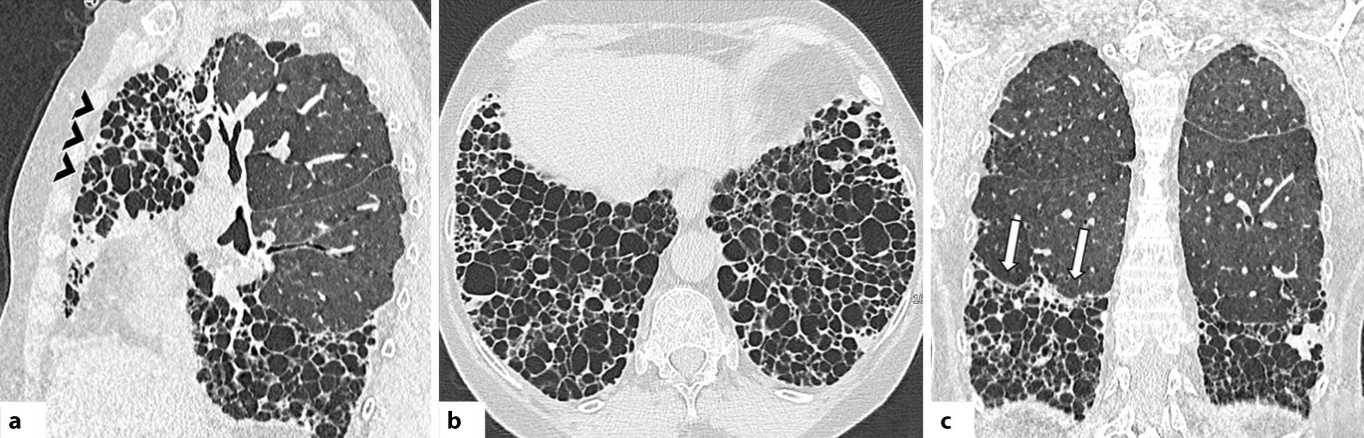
Hochauflösende Computertomographie (HRCT) von Patienten mit rheumatoider Arthritis und interstitieller Lungenerkrankung (ILD; Muster einer gewöhnlichen interstitiellen Pneumonie [UIP]), die drei für CTD-UIP typische Zeichen zeigen. a Sagittale Rekonstruktion: „anterior upper lobe sign“ (*schwarze Pfeilköpfe*) – Fibrose betont in den vorderen Anteilen der Oberlappen. b Axiale Rekonstruktion: „exuberant honeycombing“ – vergrößerte Zysten mit ausgedehntem Honeycombing. c Koronale Rekonstruktion: „straight edge sign“ (*weiße Pfeile*) – Fibrose überwiegend basal betont mit scharfer horizontaler Abgrenzung zum normalen Lungengewebe
